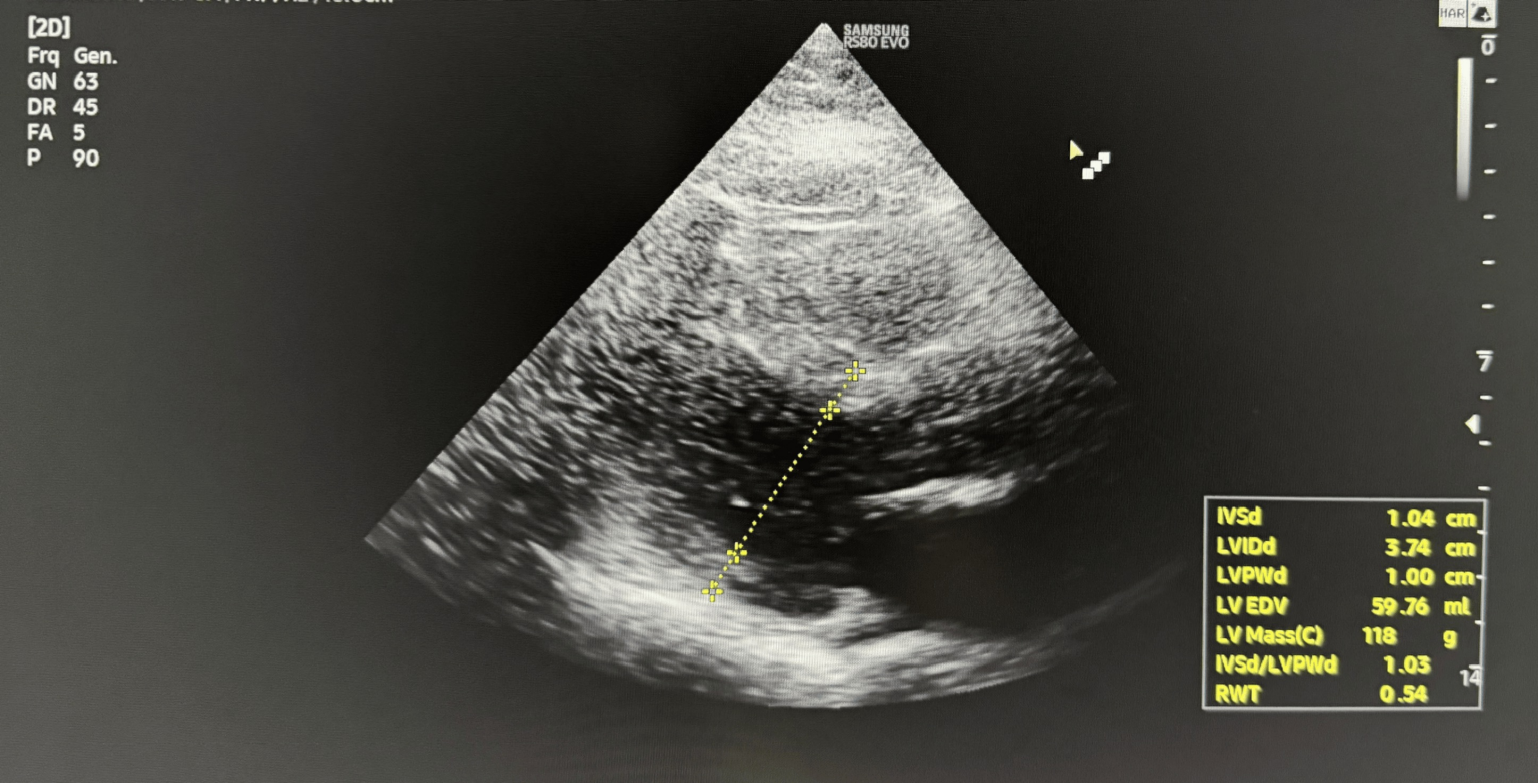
Echocardiogram showing severe global left ventricular dysfunction
